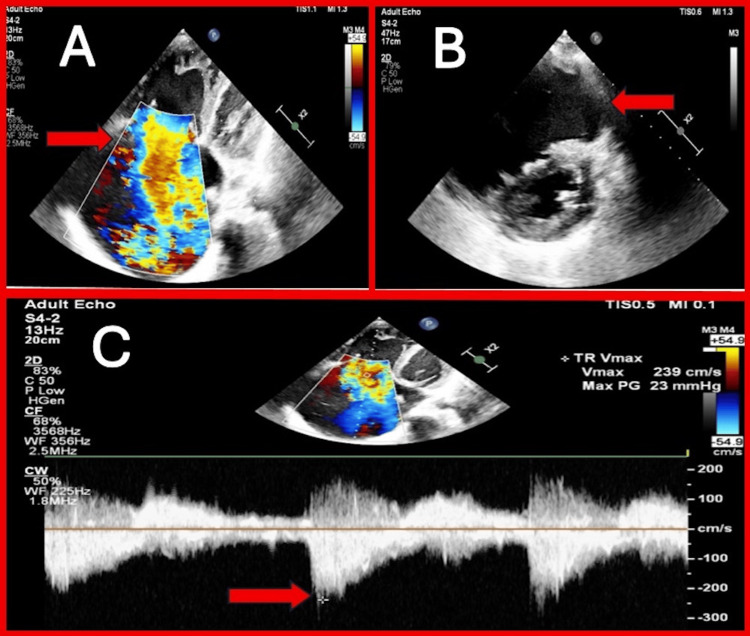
Echocardiographic findings in end-stage primary TR Panel A shows an apical four-chamber transthoracic echocardiogram with color Doppler demonstrating torrential TR. The red arrow highlights a broad systolic regurgitant jet extending from the right ventricle into the massively dilated right atrium. Panel B shows a parasternal short-axis view demonstrating severe right ventricular enlargement (red arrow). Panel C shows continuous-wave Doppler interrogation demonstrating a dense triangular regurgitant envelope with a peak velocity of 2.39 m/s (red arrow), consistent with severe TR and rapid right ventricular-right atrial pressure equalization during systole. TR, tricuspid regurgitation
